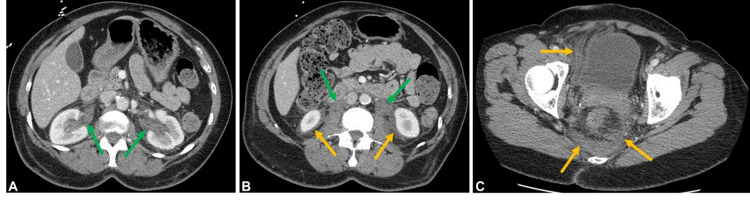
Axial contrast-enhanced CT of the abdomen and pelvis in the portal venous phase through the interpolar kidneys (A), lower pole kidneys (B), and through the bladder and pelvis (C) demonstrates marked and hyperdense circumferential thickening of both renal pelves extending to the ureters (green arrows). There is also hyperdense fluid consistent with hemorrhage in the bilateral perinephric regions and retroperitoneum, tracking down around the bladder and in the presacral region within the pelvis (orange arrows). The abdominal aorta appears intact.
